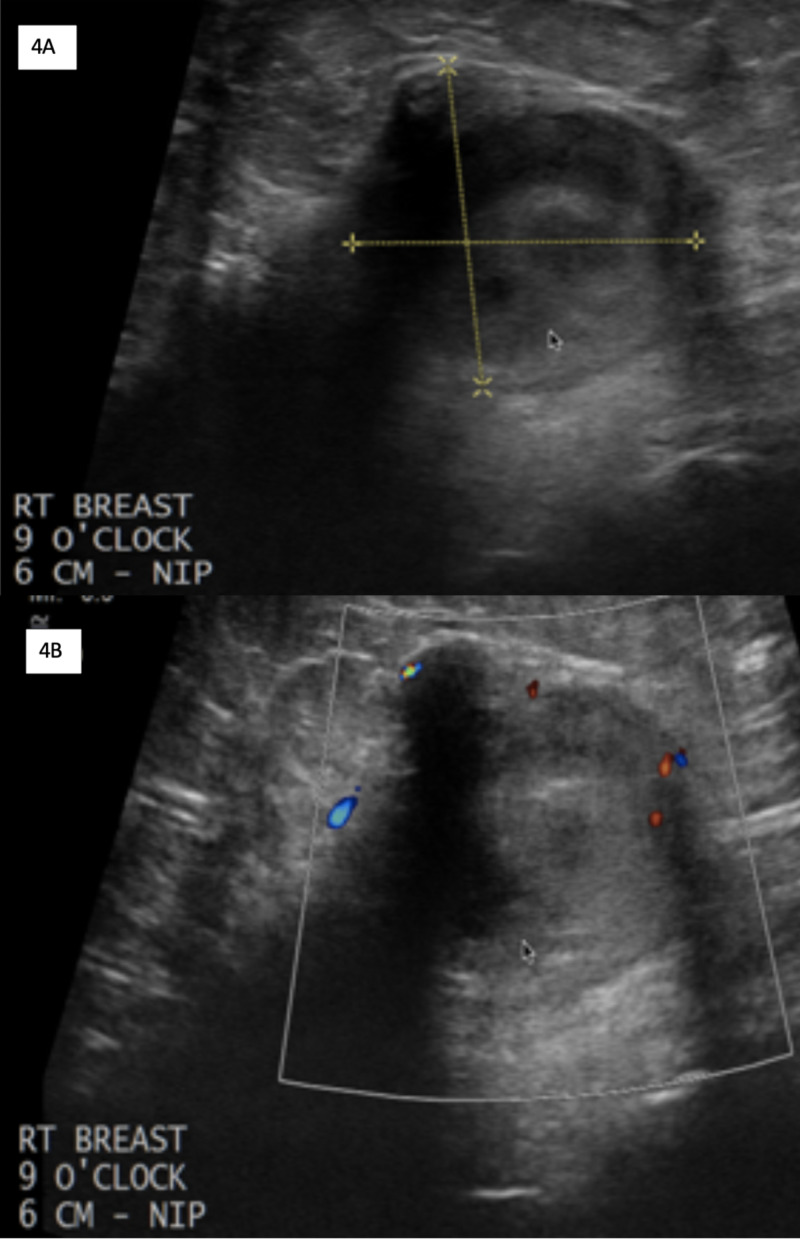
Ultrasound of the right breast Images demonstrate a circumscribed, heterogeneous mass (measured by yellow lines, A) at 9 o’clock, 6 cm from nipple, measuring 21 x 20 x 25 mm (A) with minimal color. Image B displays Doppler flow.
